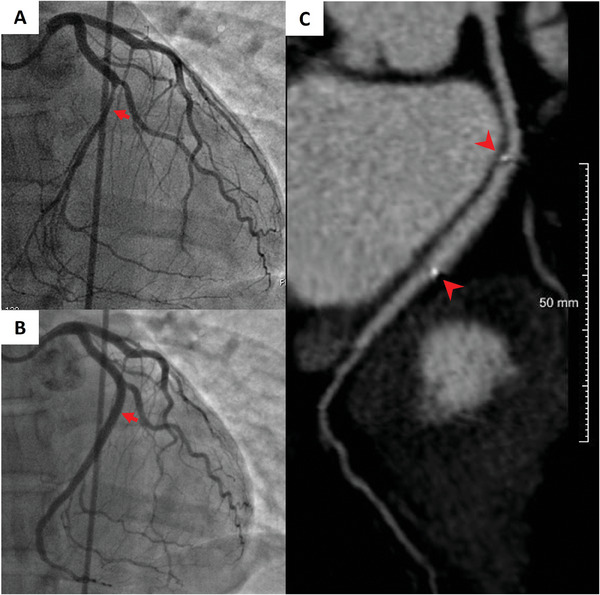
Follow‐up evaluation with CTA of Absorb BVS treatment of a spontaneous coronary dissection. Intracoronary hematoma was observed in the left circumflex coronary artery (Panel A, arrow) and treated with a BRS thereby, achieving a complete recovery of the coronary lumen (Panel B, arrow). CTA could correctly locate the previously implanted stents through the marker identification (Panel C, arrowheads) and confirm good result persistence.
